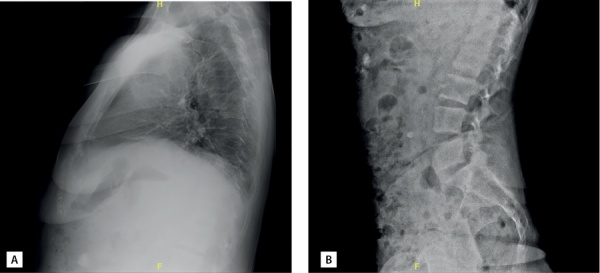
Рисунок 1. Рентгенография позвоночника пациентки В.: грудного (А) и поясничного (В) отделов в боковой проекции. Пояснения в тексте.Figure 1. X-ray of patient В’s spine: thoracic (A) and lumbar (B) sections in lateral view. Explanations in the text.
